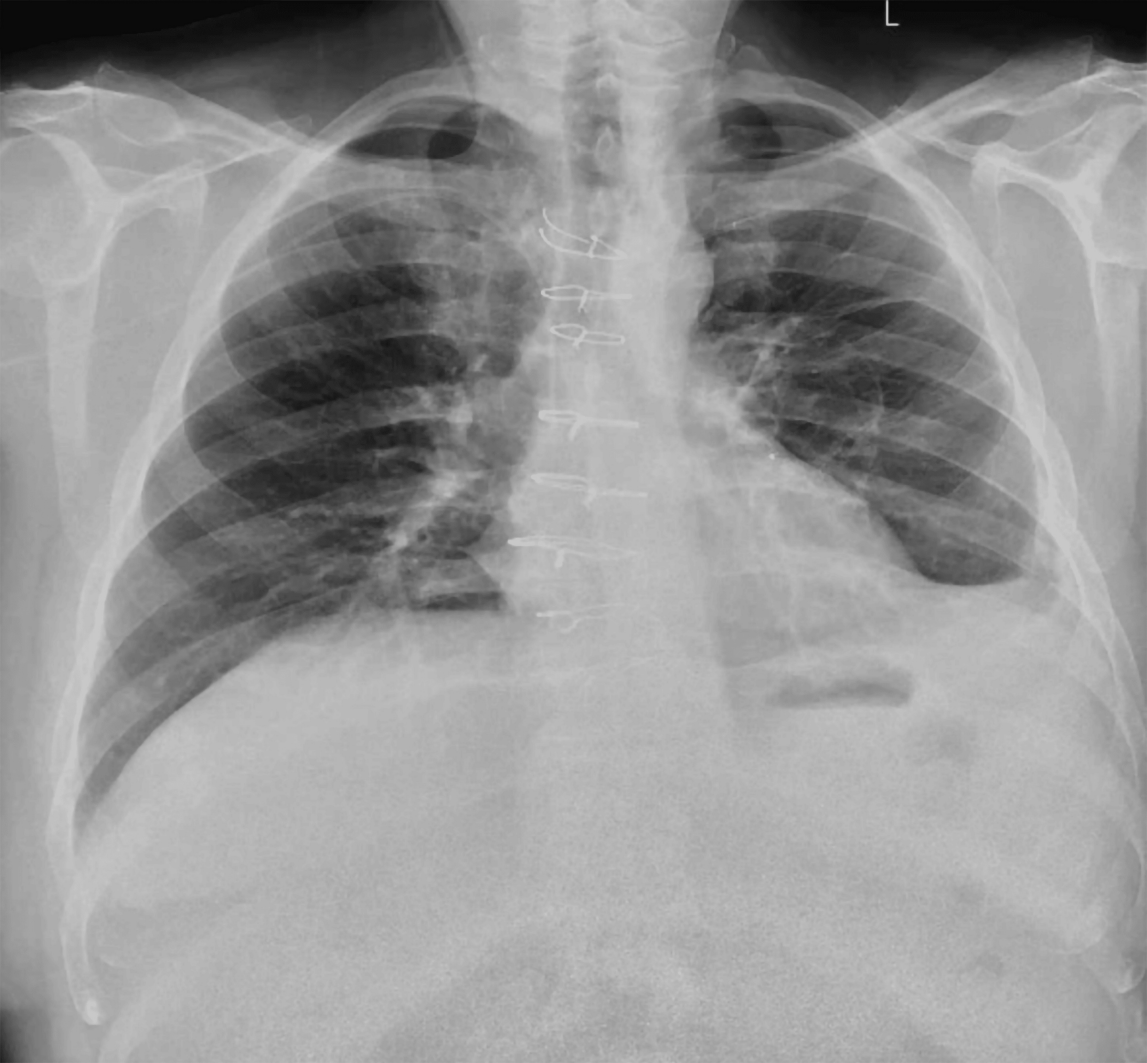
Pre-operative chest xray
